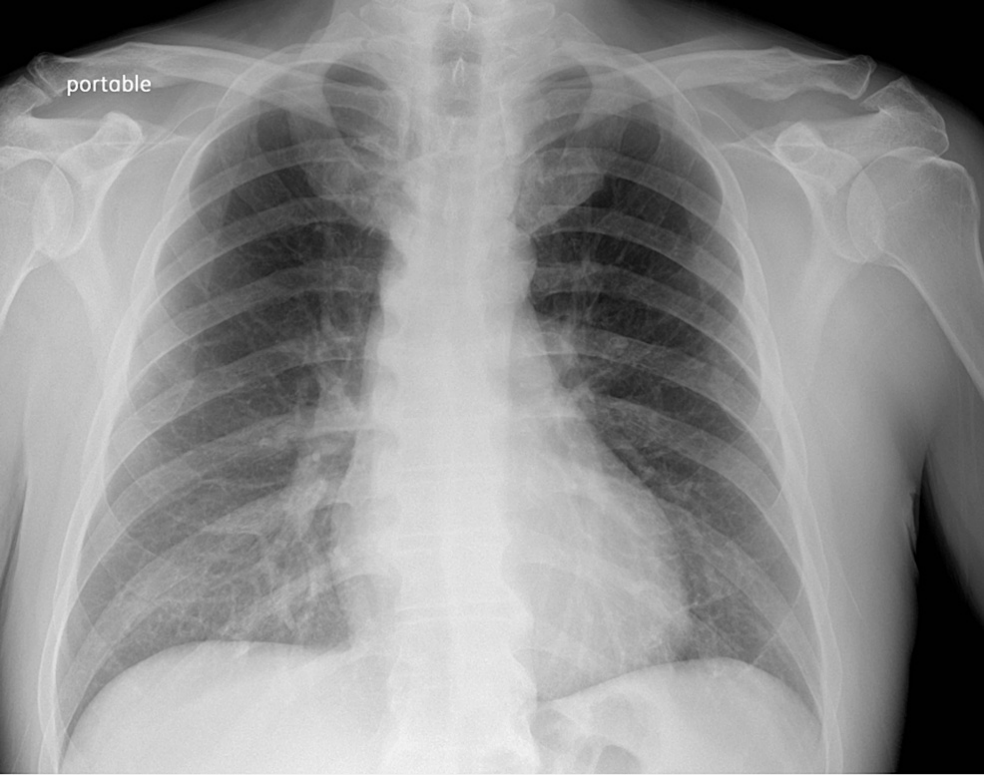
Chest X-ray The chest X-ray image shows no infiltrates or masses. No abnormality of hilar and mediastinal structures is appreciated.
